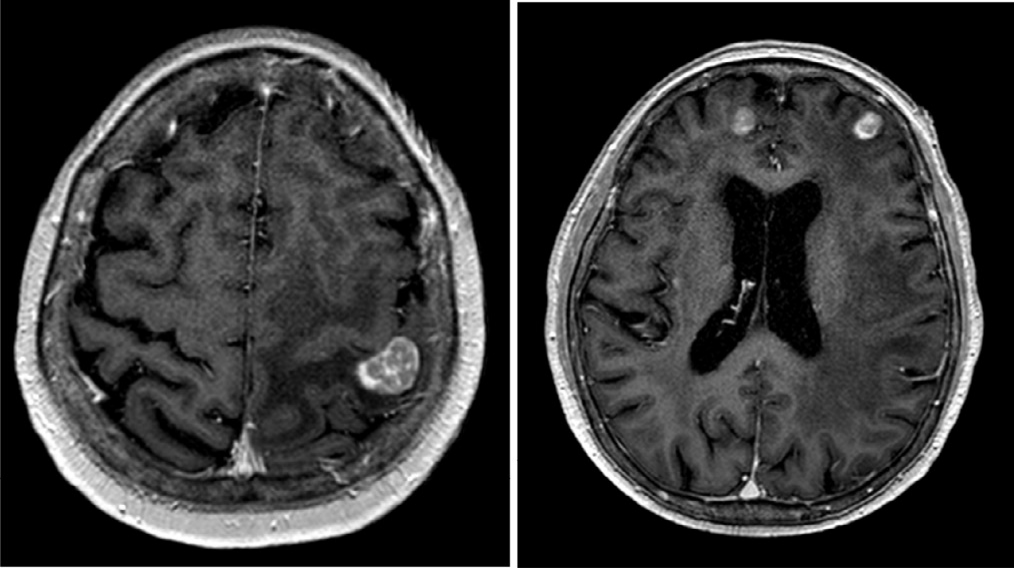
MRI brain showed contrast enhancing brain metastases in left frontal and parietal lobes. There was also another metastasis in right frontal lobe.
